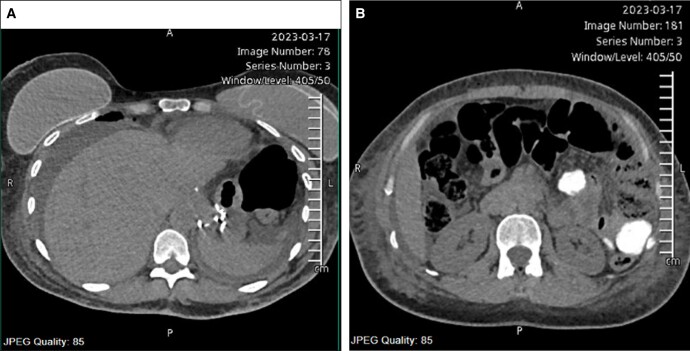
(A, B) Computed tomography scan showing active bile leak lateral to the right liver lobe.
